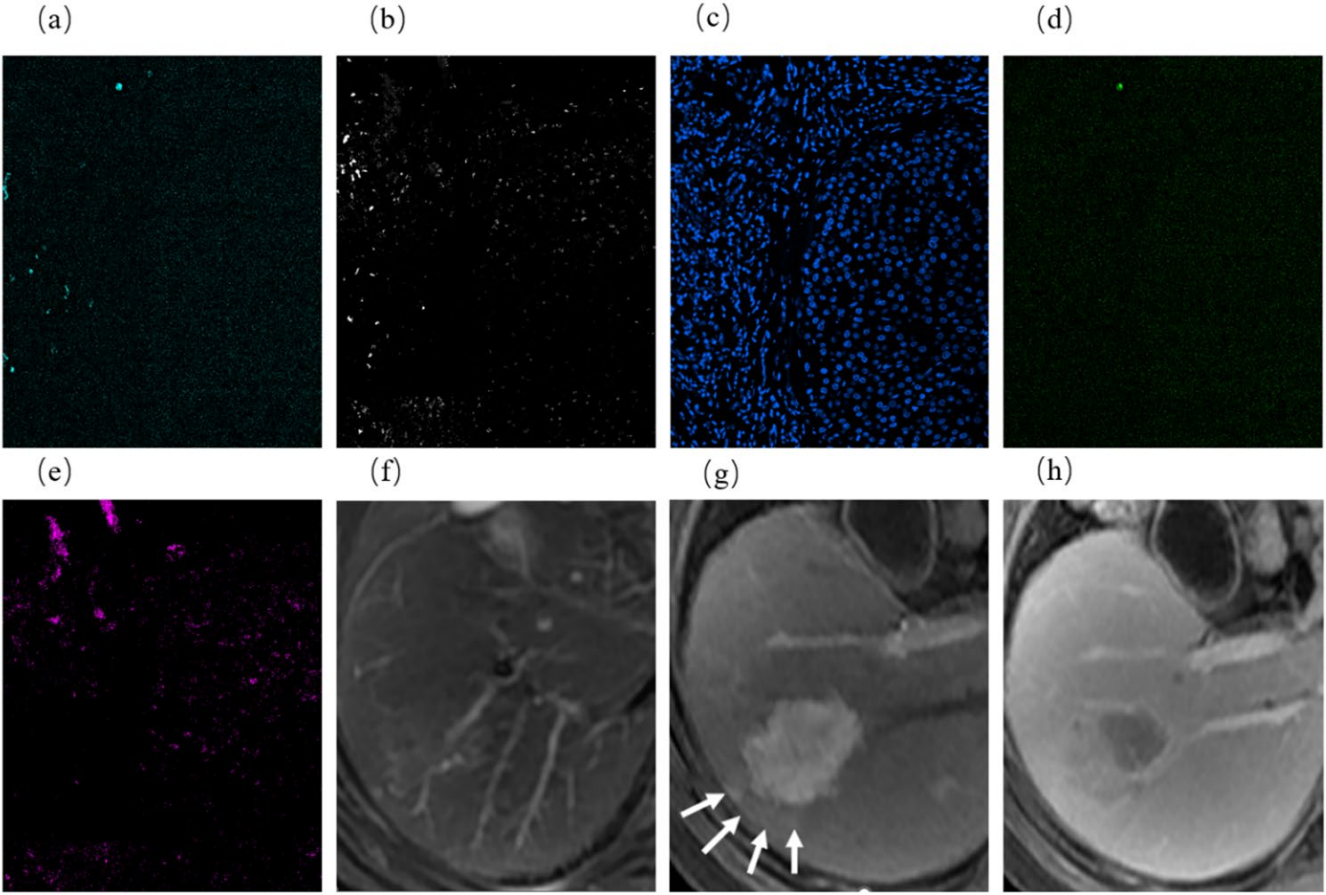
CODEX and MRI images of one patient. CODEX images: (a) CD20; (b) FOXP3; (c) DAPI; (d) Caspase-3; (e) Keratin. MRI: (f)T2WI (g) AP (h) DP. Showing a 60-year-old male with an immune score of 22, arterial peritumoral enhancement (g: white arrows), irregular shape, and no intratumoral necrosis. The patient ultimately survived for 1001 days
